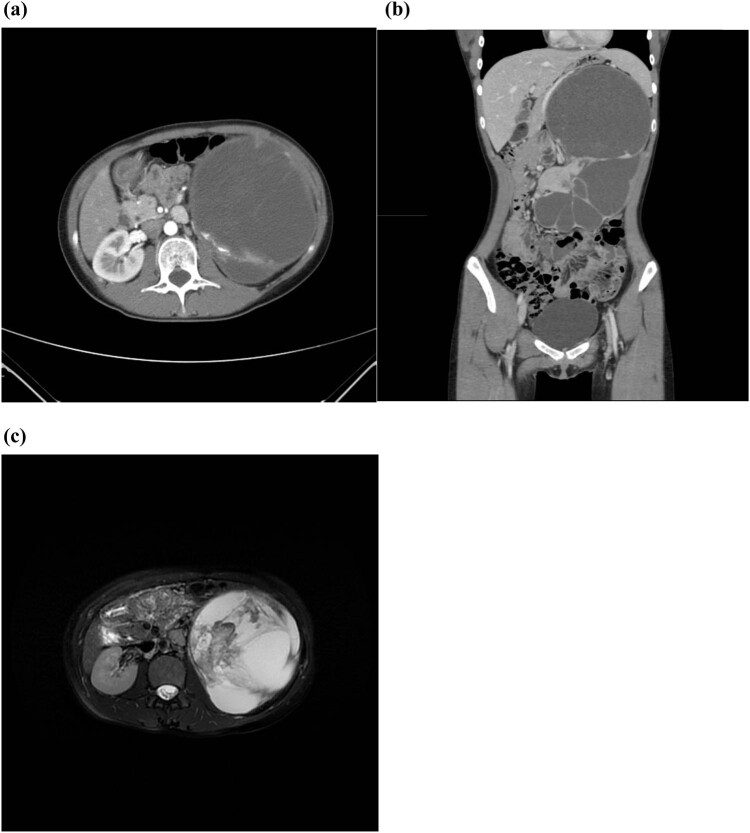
A sizeable cystic kidney with increased compartments was detected in the left abdomen on eCT and MRI scanning in 2023: (a) transverse plane, (b) coronal plane, and (c) T2 scan.
